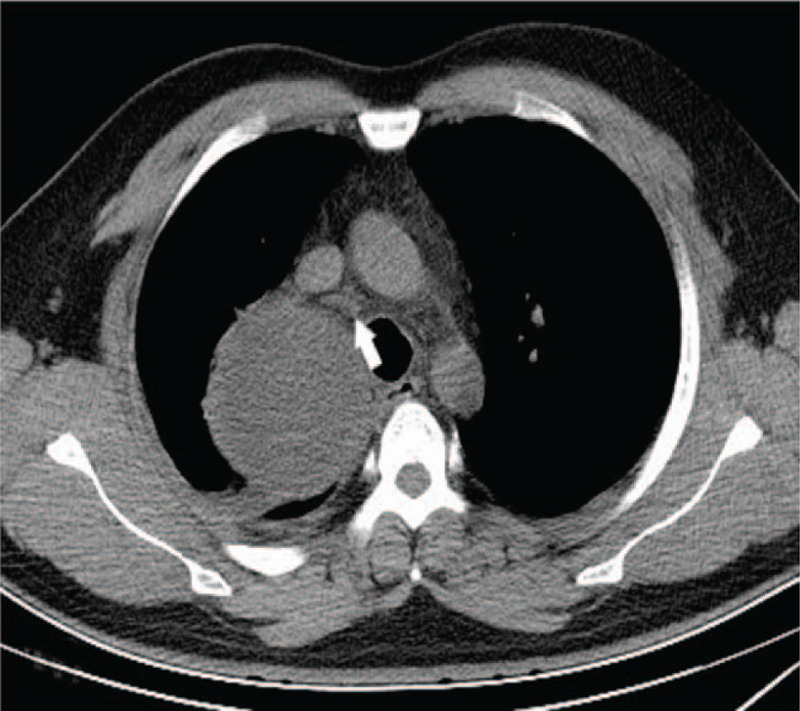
Chest CT on June 13 showed mediastinitis (arrows showing the higher mediastinal fat density).
